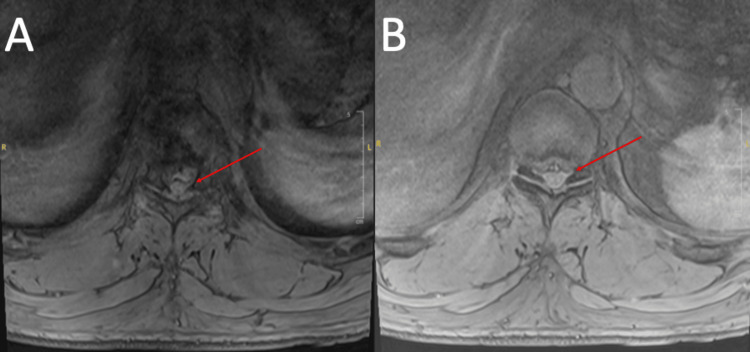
T2 axial thoracic spine MRI. (A) T9/T10. (B) T10/T11. Red arrows indicate the area of interest.
